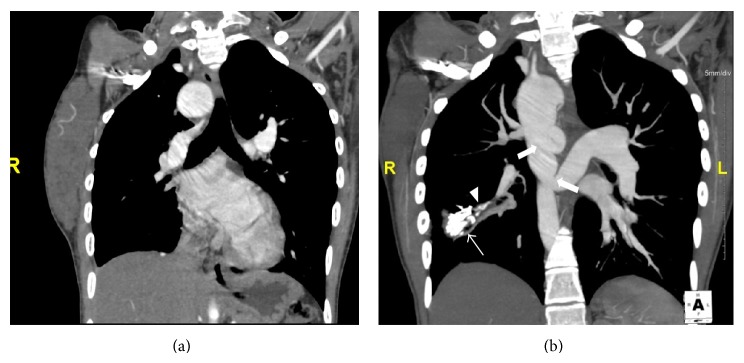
(a) and (b) Coronal CT pulmonary angiography: MIP (maximum intensity projection) images reveal a right sided aortic arch with large arterial channels arising from the left lateral aspect of the descending thoracic aorta (thick arrows) representing MAPCAs supplying the pulmonary parenchyma. Also seen is a calcified lesion (thin arrow) in the lower lobe of the right lung with a vascular pedicle (arrow head).
